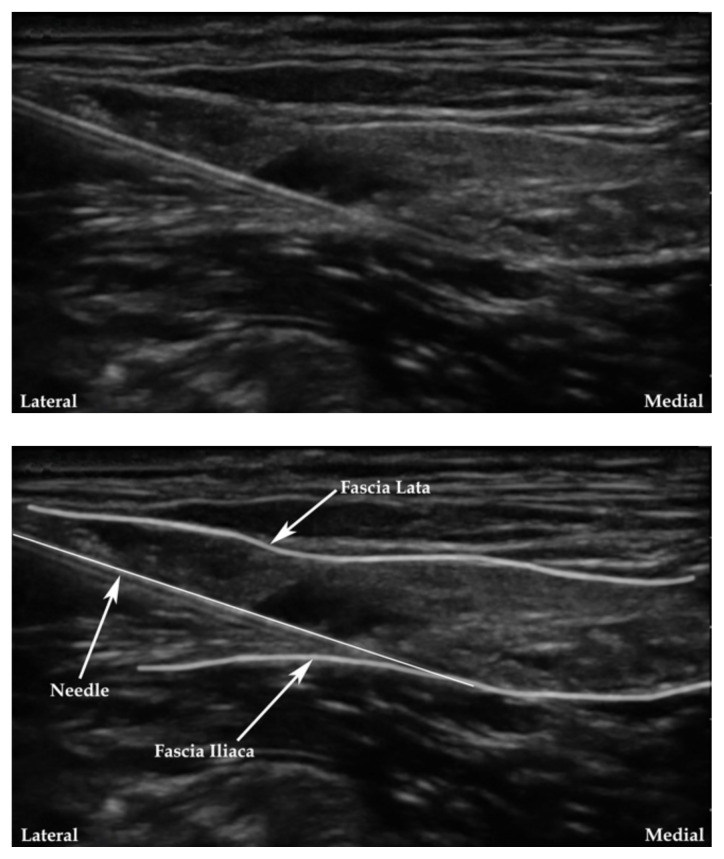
Ultrasound-guided fascia iliaca nerve block. Modified from source.^16^
